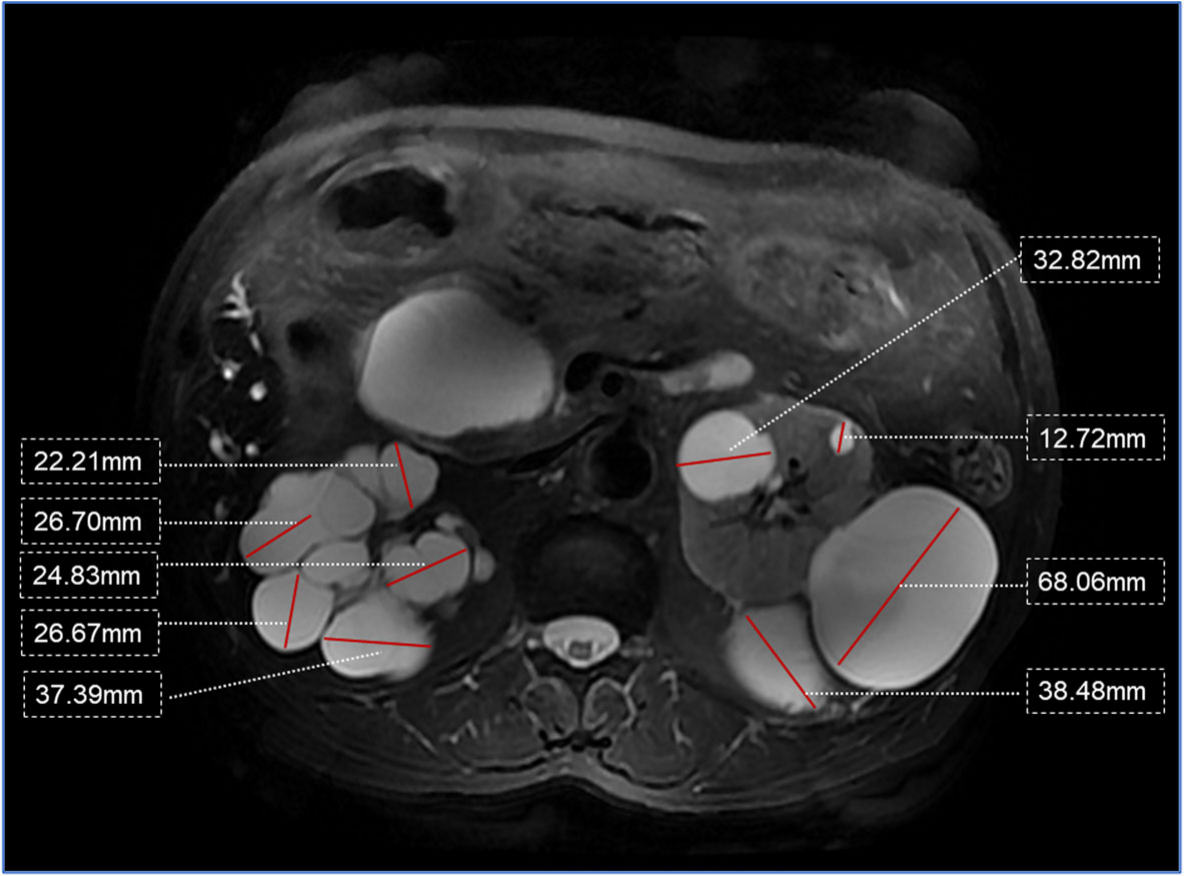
A renal MRI with 4 or more cysts with 1 cm or more in each kidney leads to the diagnosis of ADPKD
